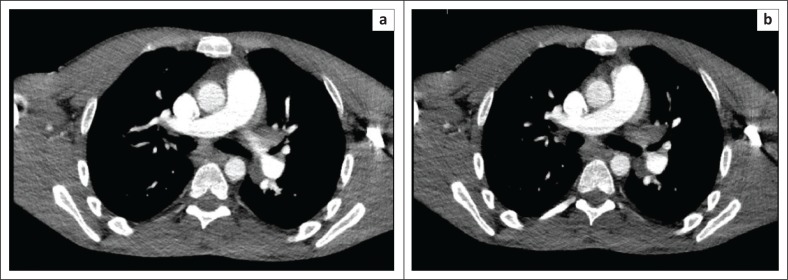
Axial slices from portal venous phase contrast enhanced CT thorax, abdomen and pelvis performed at initial presentation, which demonstrates mediastinal (a) and hilar (b) lymph node enlargement. These nodes were later not found to be FDG-avid on FDG-PET-CT.
